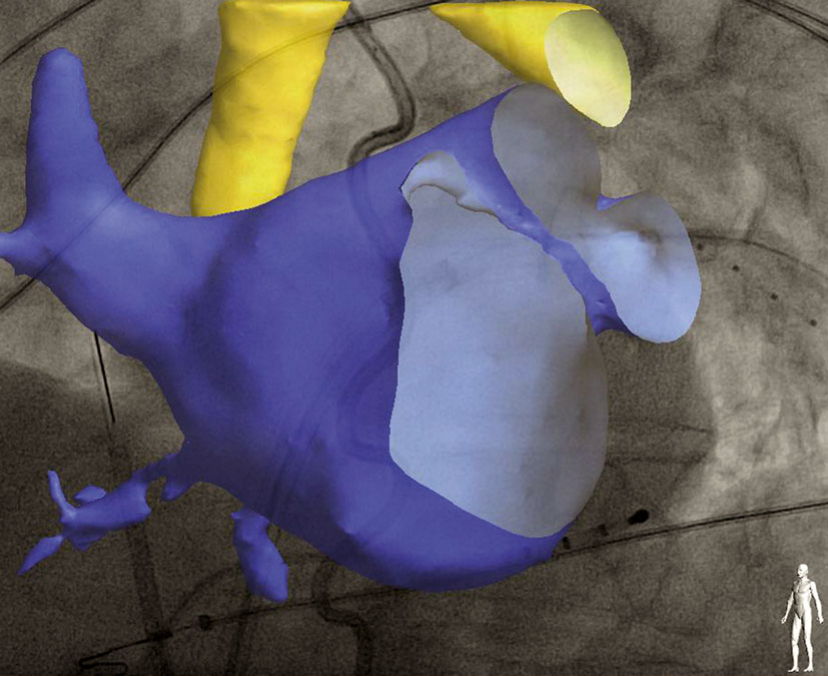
Example of a left atrial 3D CT overlay on the live fluoroscopy. The Achieve catheter is inserted into the left superior PV. A 4-pole catheter is placed in the coronary sinus
